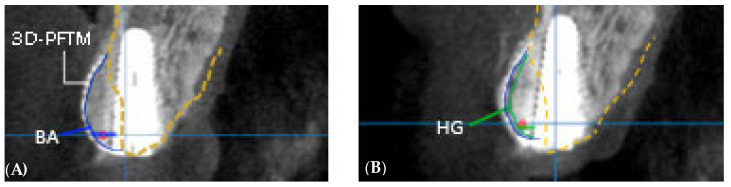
Method of radiographic analysis. A line parallel to the long major axis of the implant and a perpendicular line extending buccally at the level of the implant platform are drawn. (A) BA, bone augmentation immediately after surgery; (B) HG, hard tissue gain after 6 months of healing. The gap between the blue and green lines indicates bone resorption, while the red spot indicates the bony envelope. 3D-PFTM, three-dimensional preformed titanium mesh.
